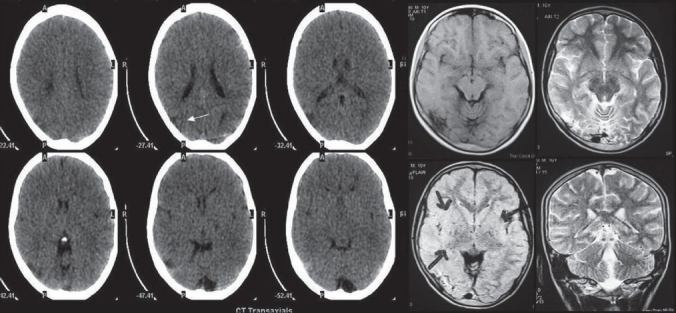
CT and MRI of the brain showing multiple lacunar infarcts bilaterally.
